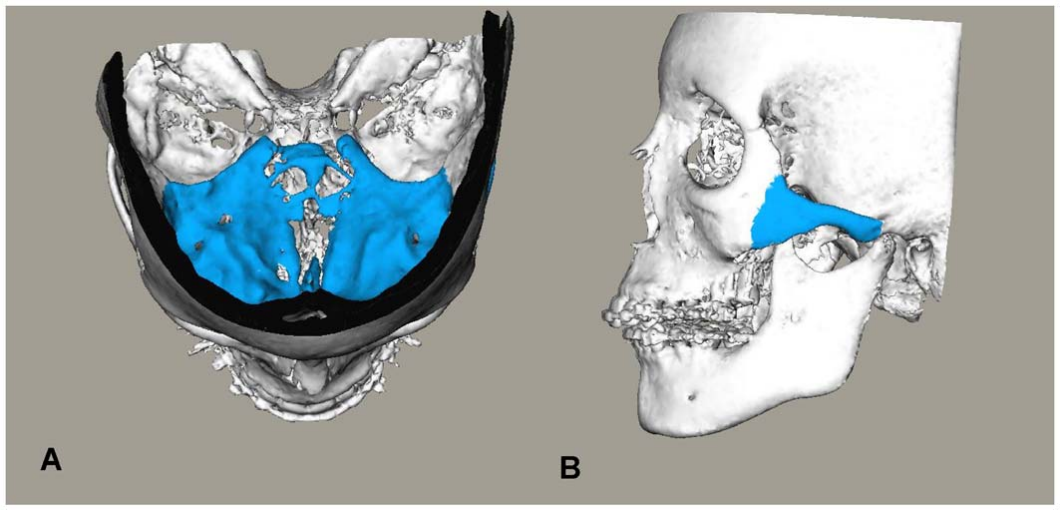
Anatomic structures used for registration highlighted on 3D CBCT models. Anatomic structures used for the registration highlighted on the 3D CBCT models. (A) Anterior cranial base. (B) left zygomatic arch.
